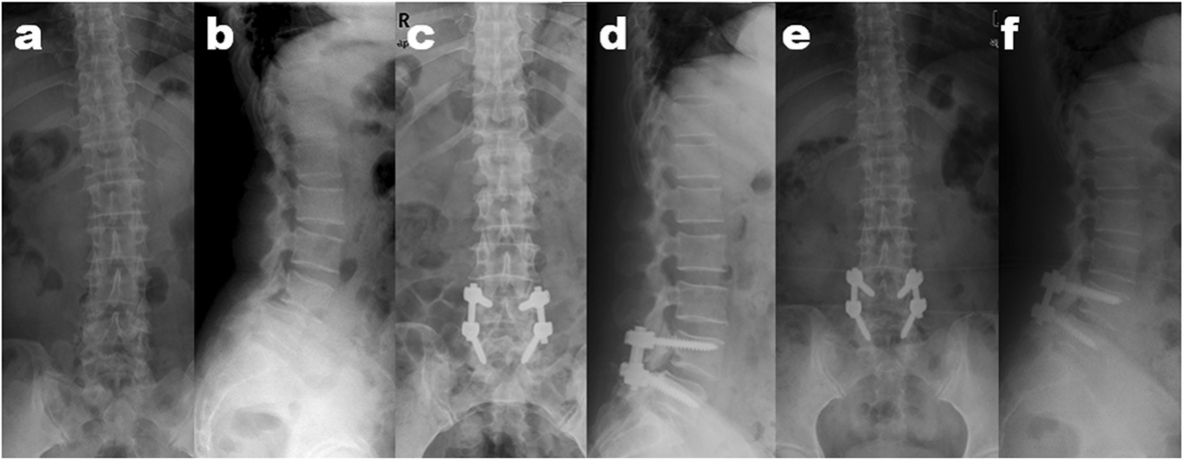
One patient with a one-level degenerative spondylolisthesis at L4–L5. (a, b) The preoperative anteroposterior (AP) and lateral radiographs of a 54-year-old woman with lumbar degenerative spondylolisthesis at L4–L5 who had severe claudicant sciatica and back pain. (c, d) Immediately after reduction surgery using lever slip reduction maneuver technique with polyaxial screw and rod fixation system through a single posterior surgical approach, AP and lateral views showed that the slip correction was 99.81%. The slip angle and the lumbar lordosis improved from 7.27° and 36° preoperation to 2.74° and 40°, respectively. (e, f) At more than 5 years follow-up, the slip correction, the slip angle, and the lumbar lordosis was 99.66%, 4.36° and 38°, respectively.
